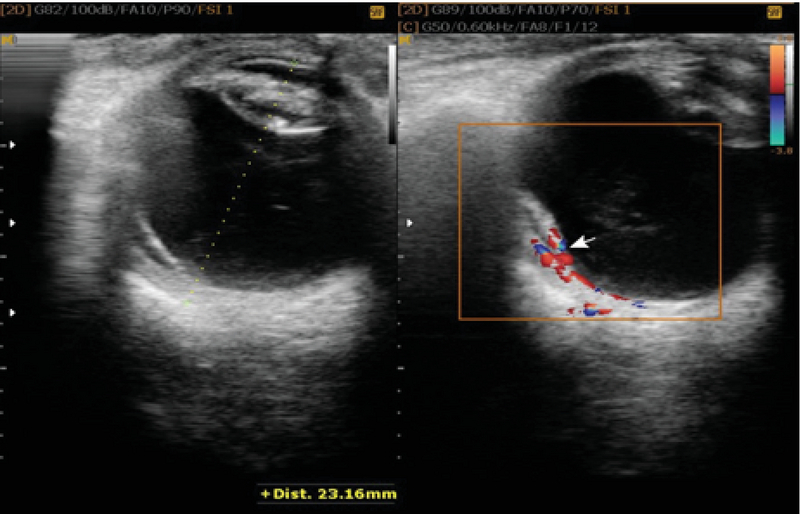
(Left) The gray-scale image of the horizontally scanned eye demonstrates central partial retinal detachment. (Right) Color Doppler ultrasound reveals thick linear and spotty color signals.
